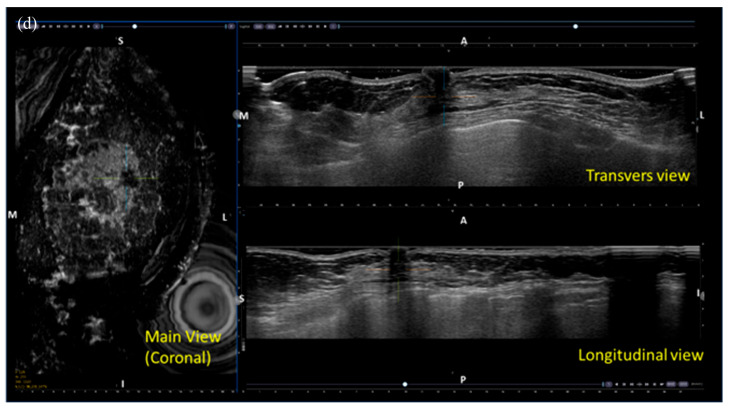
Images of the MammouS-N system: (a) Multiplanar views (axial, transverse, and longitudinal) corresponding to mediolateral, craniocaudal, and posteroanterior volumes. (b) Mediolateral oblique view: The left image shows the sagittal view corresponding to a mammographic mediolateral oblique projection. The upper-right and lower-right images represent transverse and longitudinal views, respectively. (c) Craniocaudal view: The left image shows an axial view corresponding to the mammographic craniocaudal projection. The upper-right and lower-right images show transverse and longitudinal views, respectively. (d) Posteroanterior view: The upper right image corresponds to the transverse ultrasound view, the lower right to the longitudinal view, and the left to the coronal view. Orientation markers are shown consistently across images: A = anterior, P = posterior, M = medial, L = lateral, S = superior, and I = inferior.
